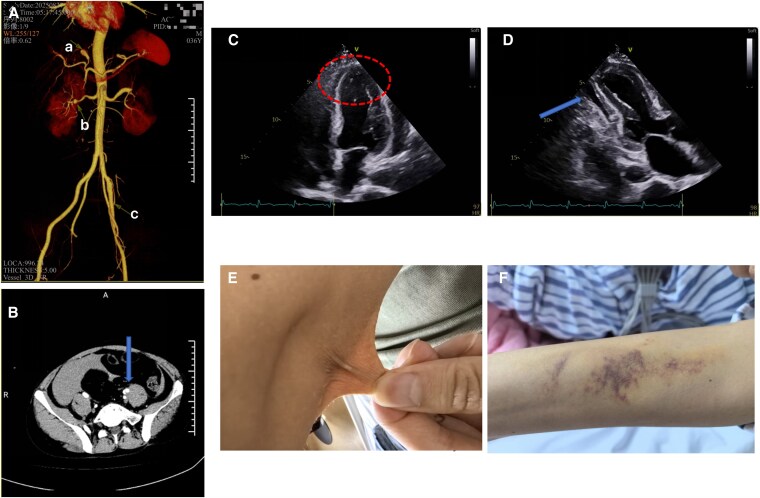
(*A*) Three-dimensional CT angiography (CTA) reconstruction demonstrating multiple vascular lesions in a patient with vascular Ehlers-Danlos syndrome (vEDS). The image shows: (*A*) hepatic artery aneurysm, (*B*) renal artery dissection, and (*C*) external iliac artery dissection. (*B*) Axial CT image showing left external iliac artery dissection (arrow) with visible intimal flap and false lumen formation. (*C*) Echocardiography shows mild-moderate apical ballooning (circled area). (*D*) Pericardial effusion (arrow) and lateral wall desynchrony. (*E*) A 36-year-old man demonstrating skin hyperextensibility upon manual traction. (*F*) This picture showed spontaneous bruising on the left forearm.
